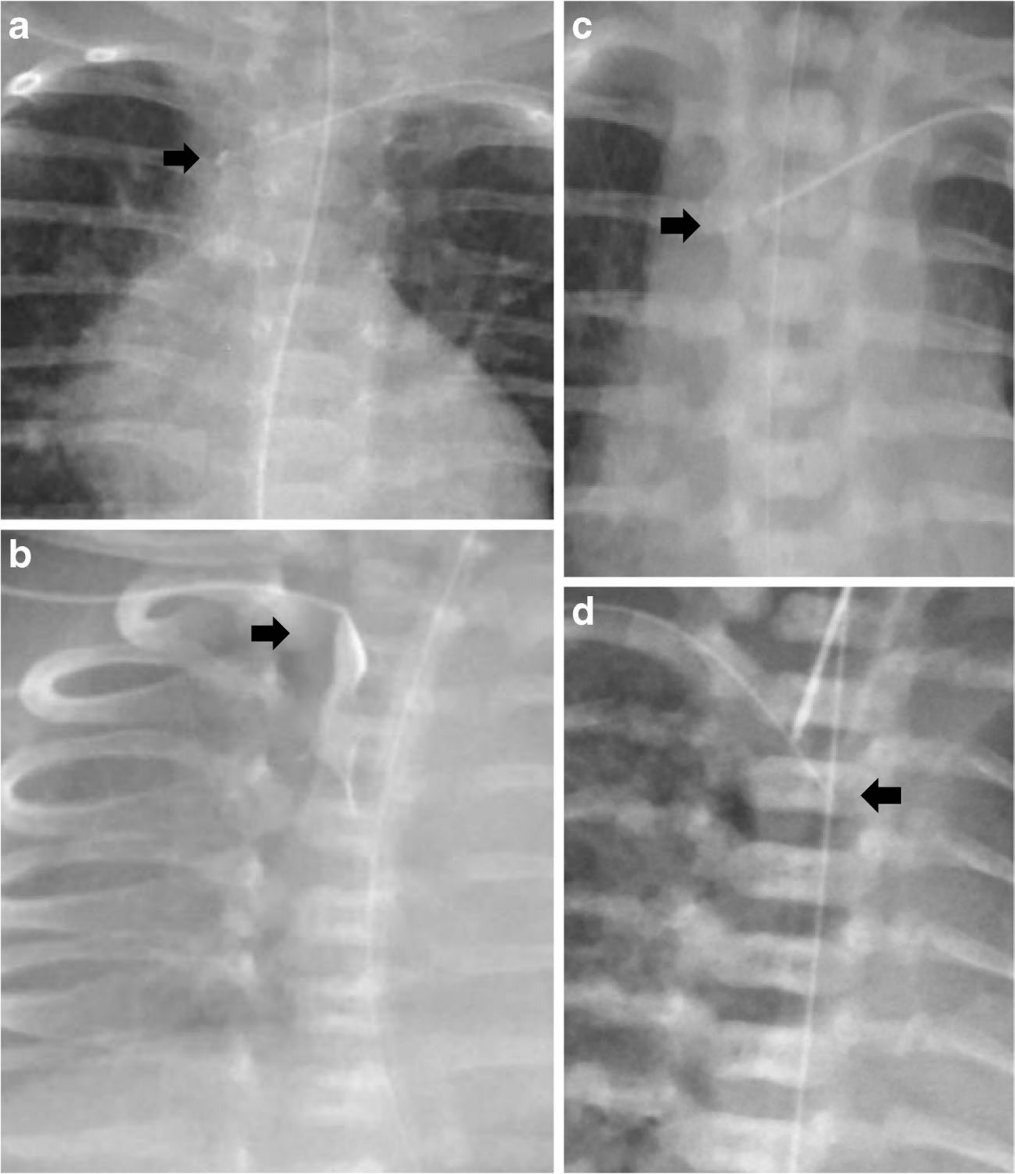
Visualization of 1 and 2 French (Fr) catheter tips with (a and b) and without (c and d) using a radiopaque agent. a A 4-day-old boy. Anteroposterior (AP) chest radiograph shows a 1 Fr peripherally inserted central catheter (PICC) with radiopaque agent (*arrow*). b A 10-day-old girl. AP chest radiograph shows a 2 Fr PICC with radiopaque agent (*arrow*). c A 4-week-old boy. AP chest radiograph shows a 1 Fr PICC without radiopaque agent (*arrow*). d A 5-week-old boy. AP chest radiograph shows a 2 Fr PICC without radiopaque agent (*arrow*)
